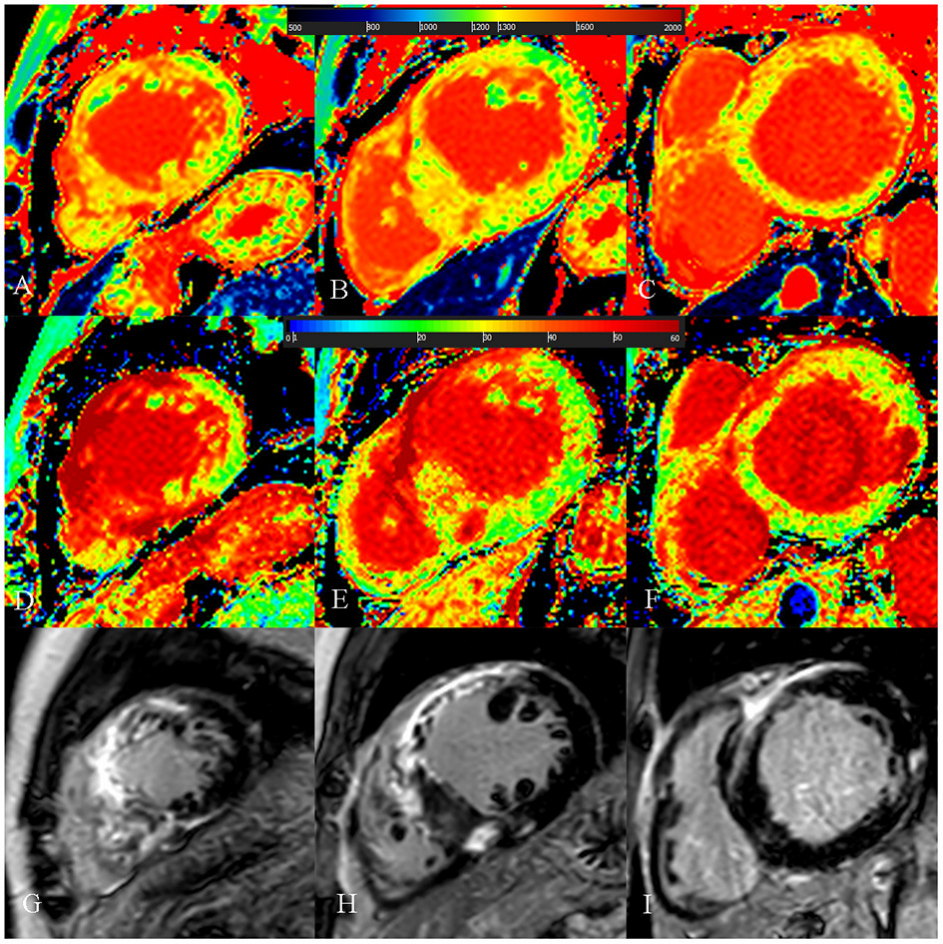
A 54-year-old male with diffuse septum hypertrophic cardiomyopathy. (A–C) Native T1 mapping of the apical, mid-, and basal portions of the left ventricular (LV) myocardium revealed higher global native T1 (1,359.67 ms). (D–F) Extracellular volume fraction (ECV) of the apical, mid-, and basal portions of the LV myocardium showed higher ECV (38.9%). (G–I) Late gadolinium enhancement (LGE) images of the apical, mid-, and basal portions of the LV myocardium showed multiple LGE. The maximal LV wall thickness was 24.3 mm, left atrial diameter was 55 mm, and the LV outflow tract gradient pressure was normal. He had non-sustained ventricular tachycardia but no family history of sudden cardiac death (SCD) and unexplained syncope. He had LGE/LV mass ≥15% (LGE/LV mass: 46.3%) and end-stage systolic dysfunction but no apical aneurysm. He was stratified as having high SCD risk under the 2014 European Society of Cardiology guidelines (5-year SCD probability: 5.93%) and enhanced American College of Cardiology/American Heart Association guidelines. He underwent the placement of ICD 6 months after cardiac MRI examinations. Higher global native T1 mapping and ECV values also indicated poor outcome.
